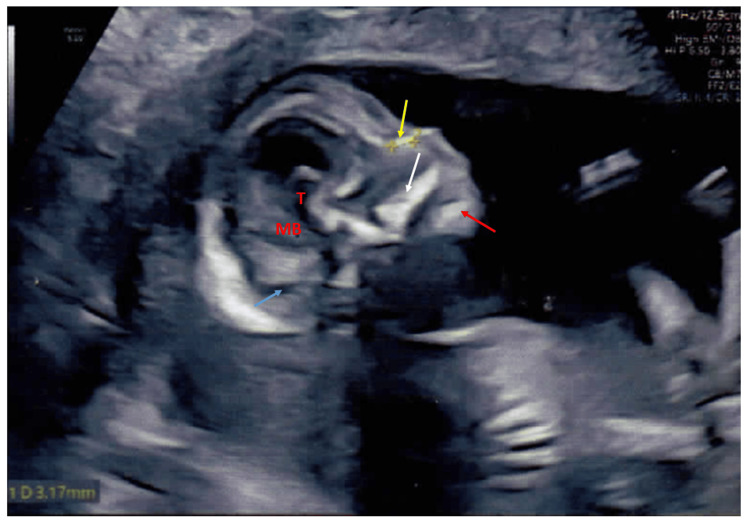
Antenatal ultrasound in the couple's second pregnancy around 15 weeks 3 days of gestation Antenatal ultrasound in the couple's second pregnancy around 15 weeks 3 days of gestation with a sagittal view of the skull with normal intracranial anatomy. Normal nasal bone (yellow arrow) measuring 3.17 mm, maxillary bone (white arrow), mandible (red arrow), normal thalamus (T), midbrain (MB), and normal cisterna magna (blue arrow) are seen.
